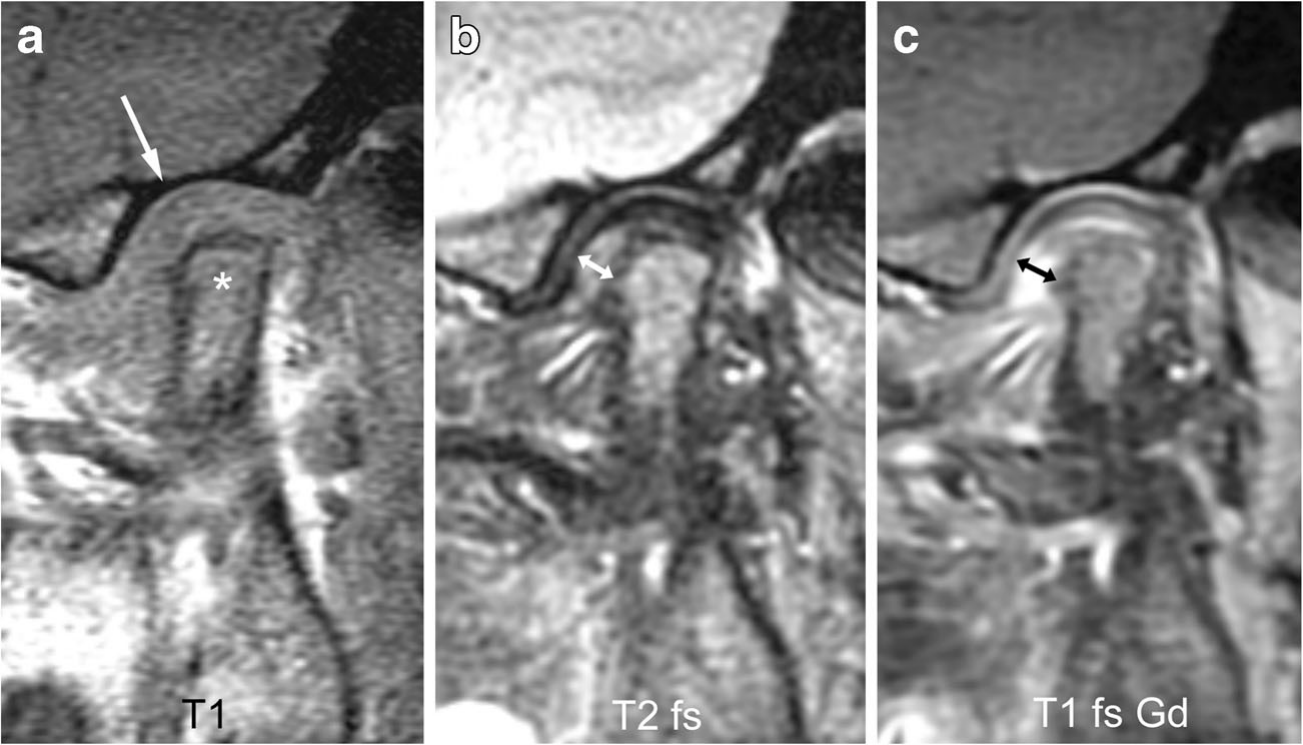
Severe synovial thickening (grade 2) and severe joint enhancement (grade 2) on sagittal oblique images in a 11-year-old girl. a There is moderate flattening of the condyle (*) and mild flattening of the temporal bone (arrow) on T1-weighted image (TR/TE 300/4.2 ms, flip angle 80°). b Thickened synovium (arrow) is shown in the anterior inferior portion of the joint space as intermediate signal intensity tissue exceeding 2-mm width on fat saturated T2-weighted fast spin echo (TR/TE 5,400/77 ms). c There is strong enhancement of the thickened synovium (arrow) but also the entire lower joint space and the posterior half of the upper joint space are shown as high signal intensity on postcontrast fat-saturated T1-weighted image (TR/TE 670/10 ms). T1 T1-weighted, T1 fs Gd contrast-enhanced fat saturated T1-weighted, T2 fs fat saturated T2-weighted
